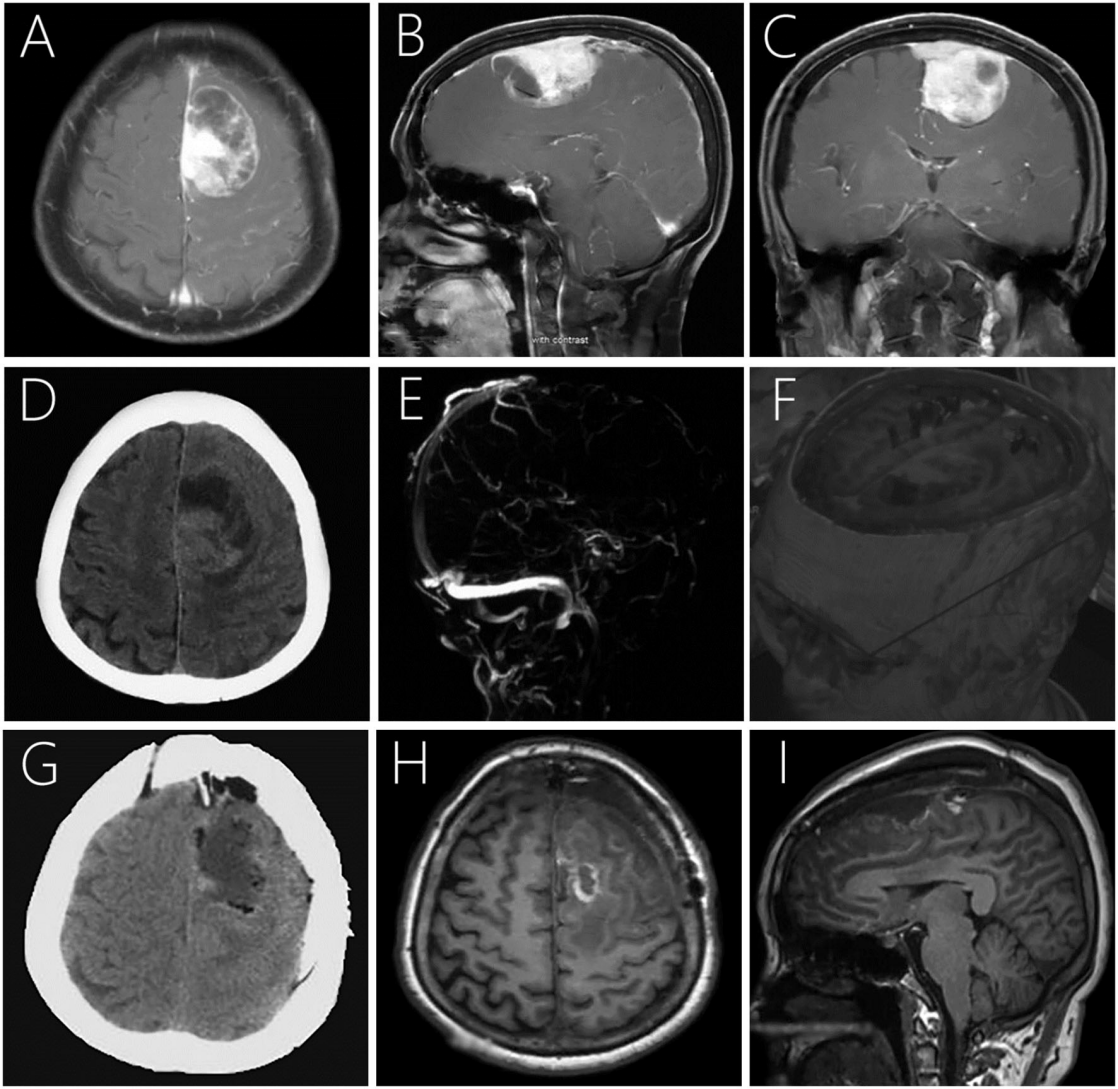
Post-contrast MRI (A–C) showing a large irregular mass with heterogeneous enhancement in the left frontal lobe with mild peritumoral edema. Plain CT demonstrating a large mass mixed hypo- and isodensity (D). Magnetic resonance venography showing no development was seen in the superior sagittal sinus of the frontal area (E). Diffusion tensor imaging showing compression, displacement, and partial interruption of nerve fibers in the lesion area (F). Postoperative CT (G) and MRI 7-days postoperatively (H,I) revealed that the lesion was completely removed with no signs of recurrence.
